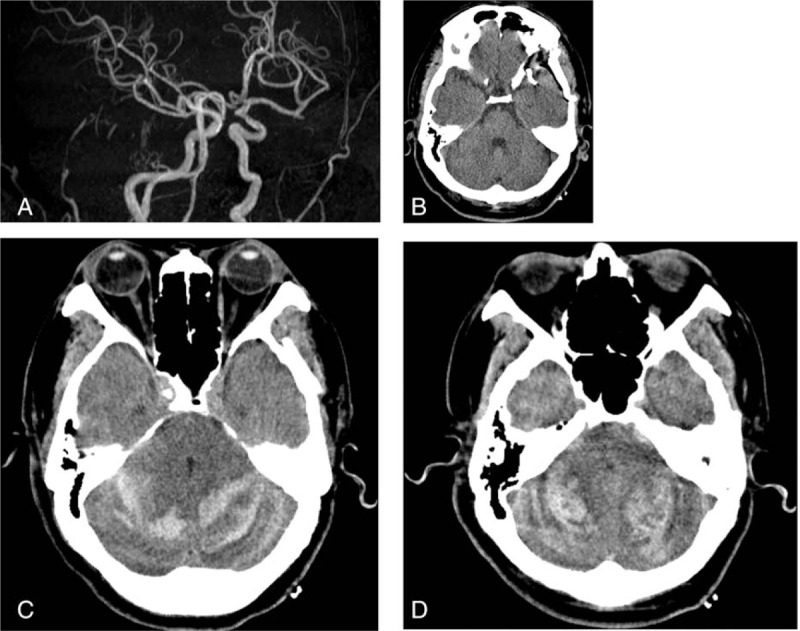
Preoperative MRA (A) shows unruptured intracranial aneurysm on left MCA bifurcation. Immediate postoperative head CT shows air density on the left frontotemporal area with a clip artifact (B). Follow-up head CT obtained 20 hours after clipping shows bilateral RCH with the characteristic zebra sign on superior folia and a massive mass effect on the fourth ventricle (C,D). CT = computed tomography, MCA = middle cerebral artery, RCH = remote cerebellar hemorrhage.
